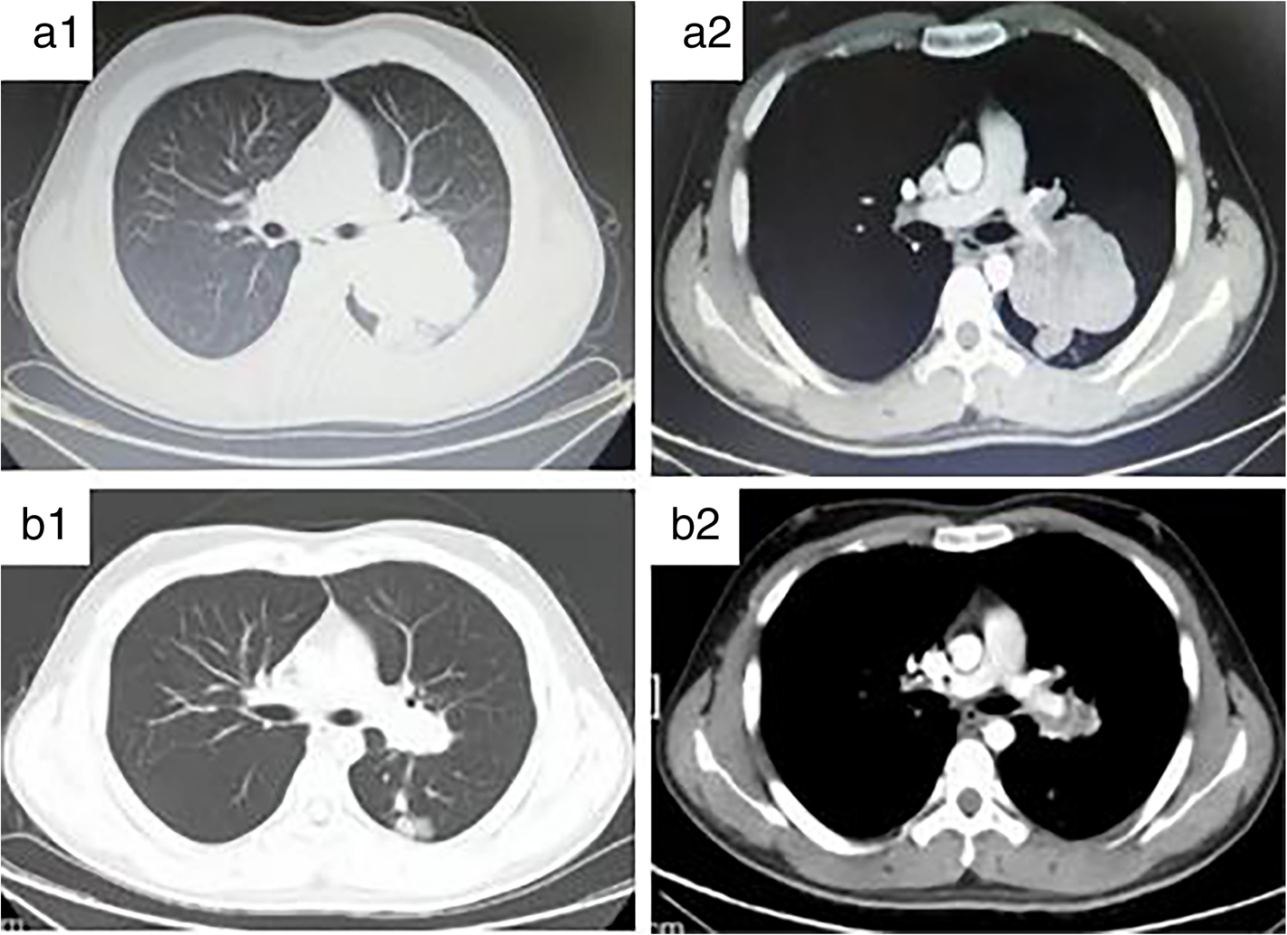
CT thorax. (a1) A large irregular and well‐defined border mass lesion measuring 7.7 × 6.3 cm beside the left hilus pulmonis and an enlarged mediastinal lymph node measuring 2.7 × 1.9 cm. (a2) The left inferior pulmonary artery is surrounded and the left pulmonary artery was squeezed and deformed. (b1) The tumor was remarkably reduced to 2.5*3.4 cm and the mediastinal lymph node was 1.2 cm. (b2) The left pulmonary artery was surrounded, which was slightly narrower
